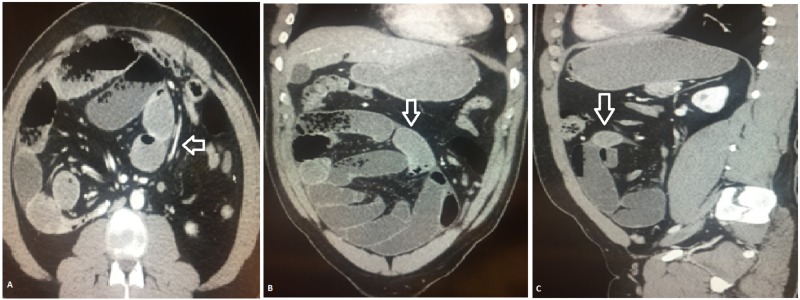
MDCT axial (A), coronal (B), and sagittal (C) images showing internal herniation of the small bowel with cocoon formation (arrow) MDCT: multidetector computed tomography
